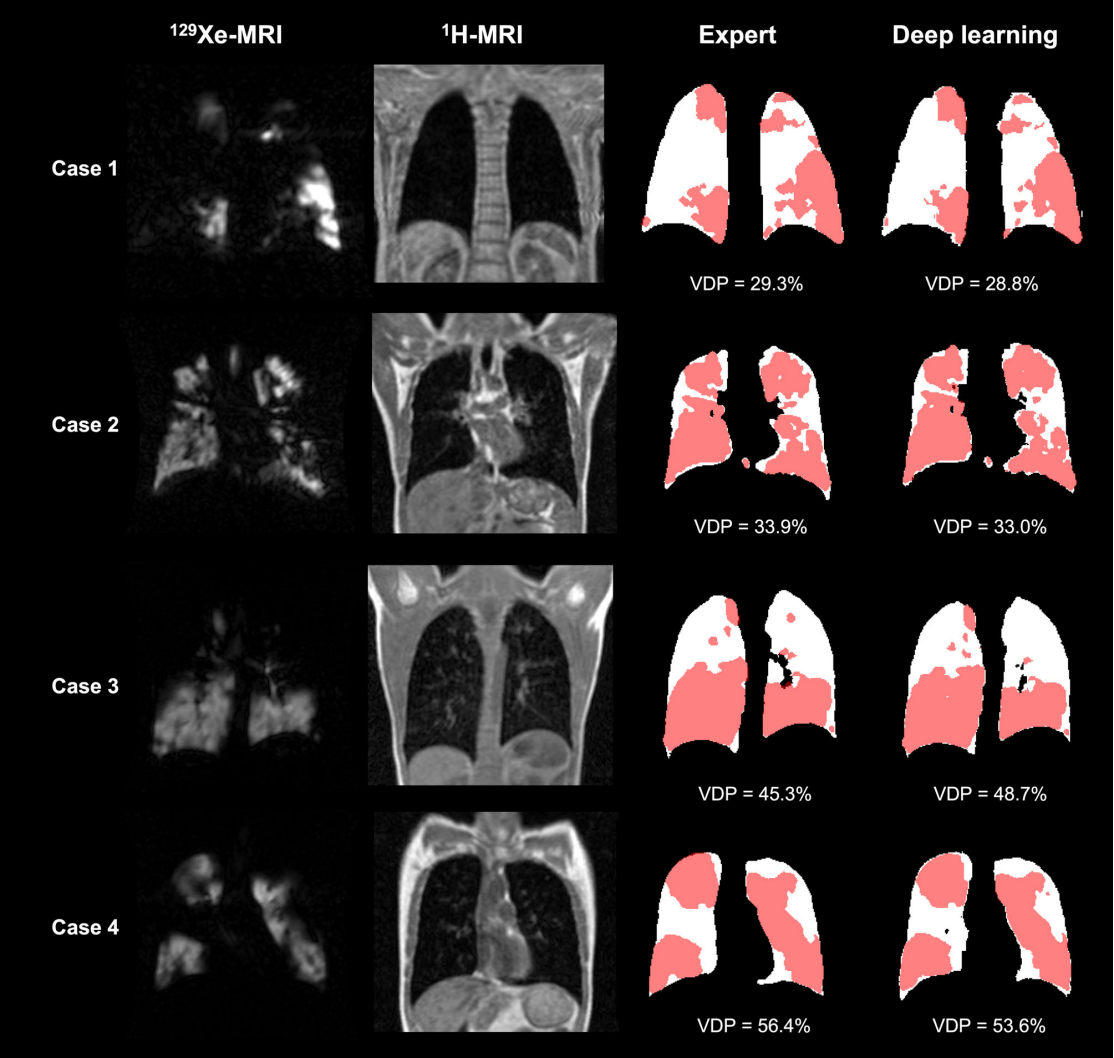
Example coronal slices of four cases with ventilation defects showing fused manual LCEs (white) and hyperpolarized gas MRI ventilated lung segmentations (pink) compared to those generated using the dual‐channel DL method and previously described hyperpolarized gas MRI ventilated lung segmentation method. Manual and DL‐generated VDPs are given for each case.
